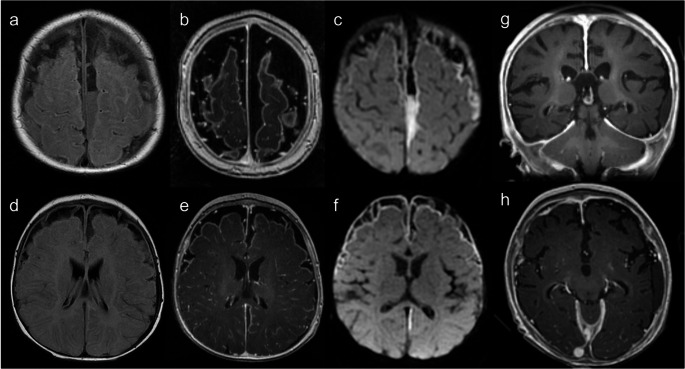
Immunodeficiency under investigation. A 3-month-old female patient with *Salmonella* meningitis. The patient has a history of neonatal sepsis without an identified causative agent and *Campylobacter jejuni* bacteremia in the context of acute gastroenteritis. Brain MRI (a and d – axial FLAIR, b and e – gadolinium-enhanced axial T1, and c and f – axial b1000 DWI) demonstrates extensive leptomeningeal involvement, with a fluid level of purulent material in the left parafalcine region, but no hydrocephalus or visible parenchymal abscesses. (g and h) Gadolinium-enhanced axial T1-weighted images 2 months after treatment show a favorable evolution of the leptomeningeal infection; however, there is generalized supra- and infratentorial pachymeningeal thickening and mild diffuse cerebral atrophy
